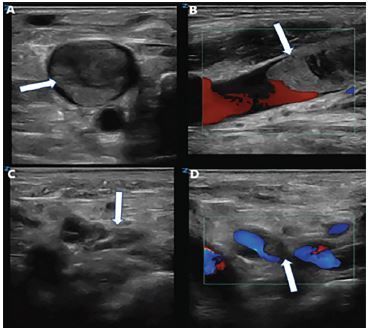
A) Thrombus in the right internal jugular vein (arrow) seen on transverse view. B) Sagittal view of right internal jugular vein thrombus (arrow) with color doppler showing obstruction of venous outflow and turbulence proximal to the thrombus. C) Thrombus in the distal left internal jugular vein (arrow) seen on transverse view. D) Color doppler of the left internal jugular vein thrombus (arrow) showing partial occlusion of the lumen seen on transverse view.
